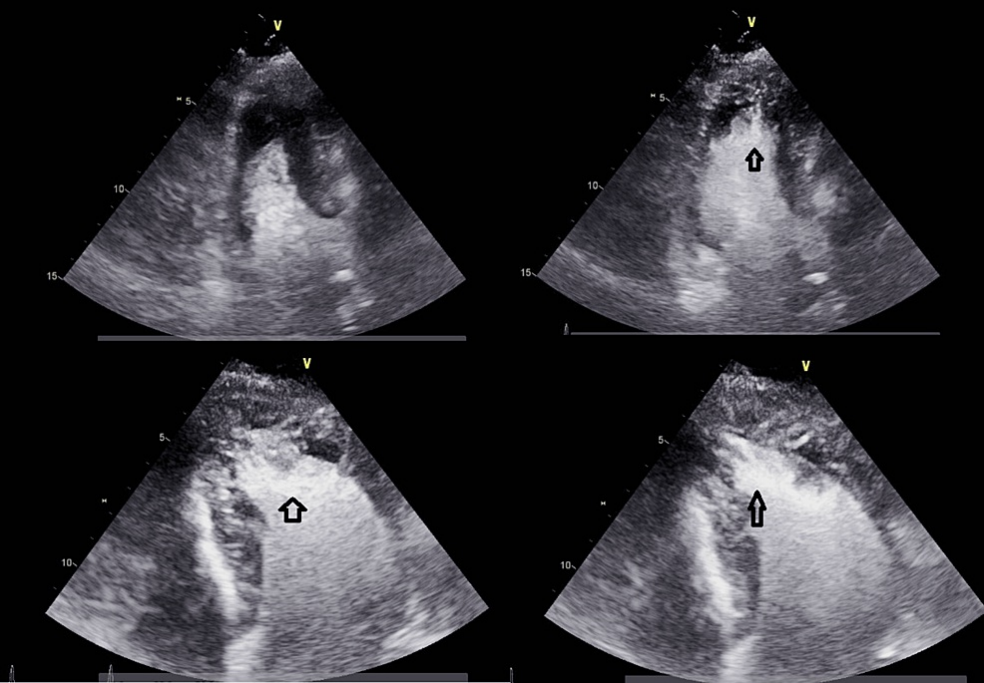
A transthoracic echocardiogram revealed mild to moderate concentric left ventricular hypertrophy with asymmetric thickening of the apical segment (black arrows).
